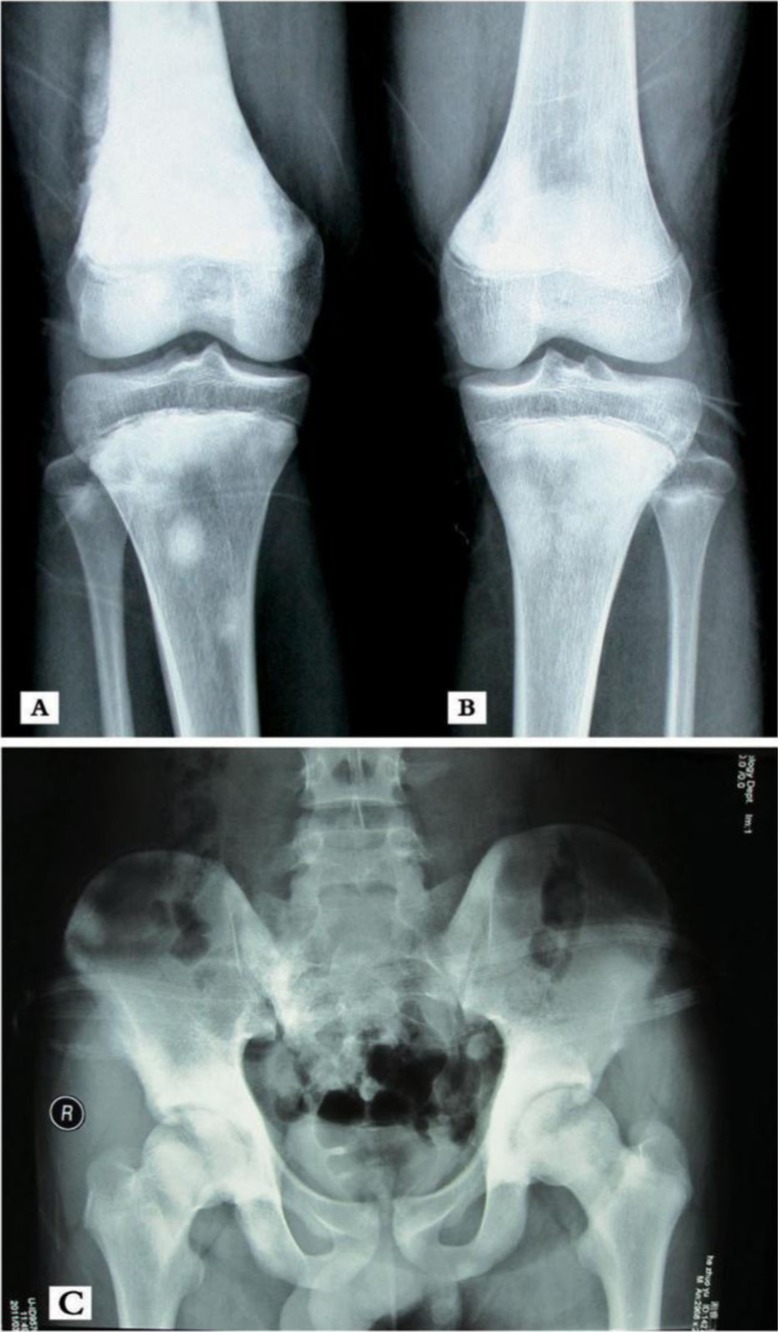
X-ray showing pure, patchy osteosclerotic lesions of (A) distal femurs, (B) proximal tibiae, and (C) hip bones, ilium, sacrum, and proximal femurs. Bony destruction is likely evident in the right distal femur and appears to be surrounded by a patchy periosteum reaction.
